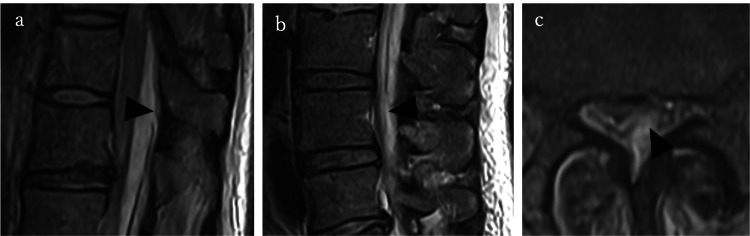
Pre-treatment MRI showing the tear in the dura mater Sagittal T2-weighted images showing (a) the cranial portion at the Th11/12 level and (b) the caudal portion at the L3/4 level. (c) Axial T2-weighted images showing the dural tear MRI: magnetic resonance imaging
